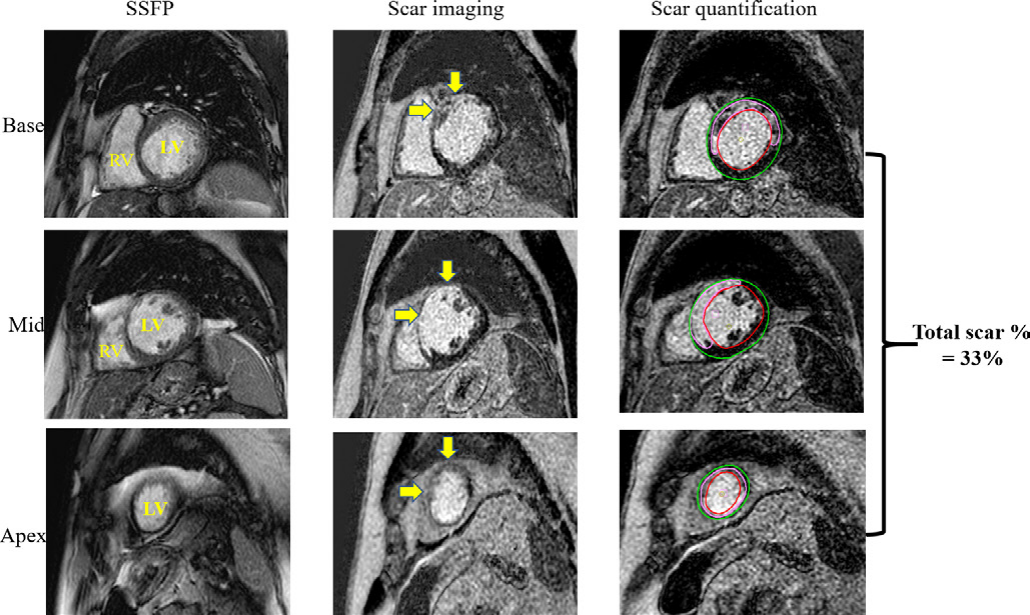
Cardiac MRI and scar quantification. Example of cardiac magnetic resonance short axis stack acquisition, scar imaging and scar quantification from a patient with prior left anterior descending artery infarction and resultant ischaemic cardiomyopathy. For presentation purposes, only three slices (one at the base, one at the mid cavity and one at the apex) are shown. The yellow arrows point to the scar. Scar quantification is performed by first defining the endocardial (in red) and epicardial (in green) contours. Scar is shown here in pink. For accurate calculation of total scar %, the entire LV stack needs to be contoured from the atrioventricular junction (mitral annulus) to the apex. LV, left ventricle; RV, right ventricle; SSFP, steady state free precession.
